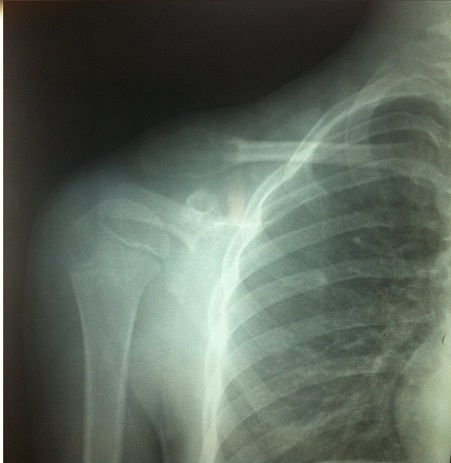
Radiographie de face de la clavicule droite montrant la lacune de l’extrémité externe
